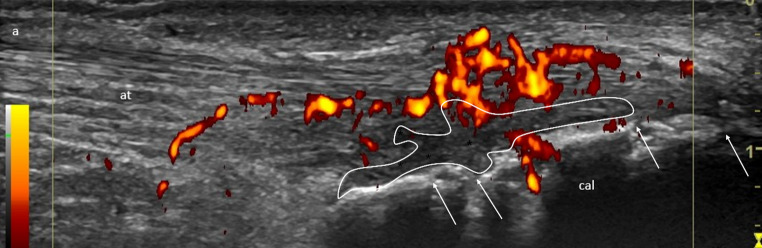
Ultrasound detected enthesitis in psoriatic arthritis. Legend. (a) Image showing active, erosive enthesitis at the calcaneal insertion of the Achilles tendon. Note the presence of entheseal thickening, hypoechoic areas (outlined by white line), and power Doppler signal at the enthesis, including filling of cortical bone erosions pointed out by arrows (dorsal longitudinal view). Acronyms: at: Achilles tendon; cal: calcaneal bone
